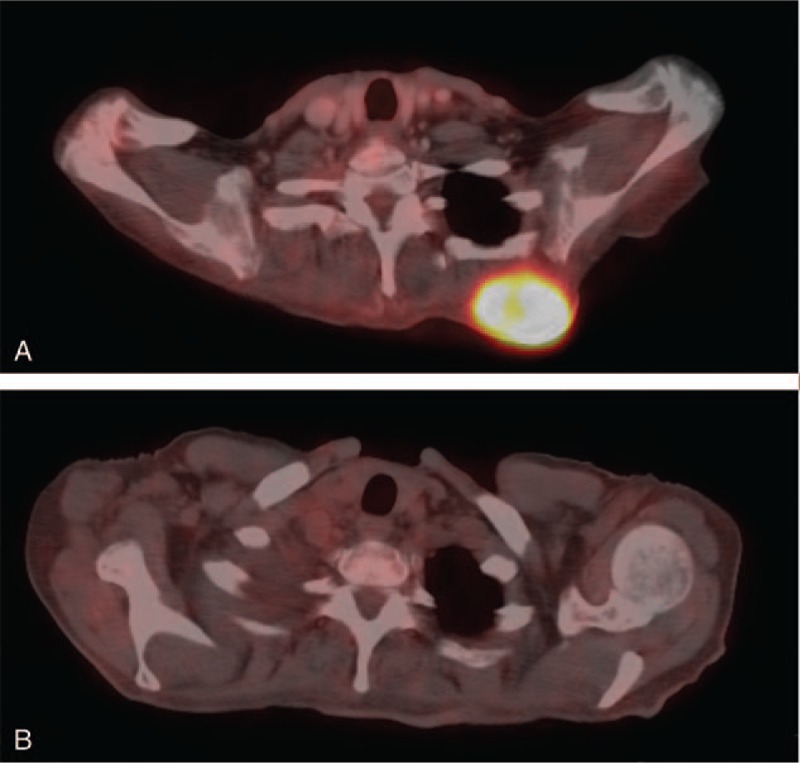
(A) PET-CT demonstrating a subcutaneous metastatic lesion, 2 months after starting nivolumab treatment (03/2015). (B) PET-CT demonstrating complete resolution of the lesion 5 months after starting nivolumab treatment (06/2015).
